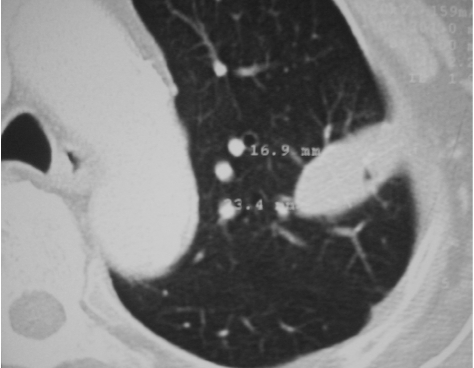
CT scan performed one-month post-RFA. This is a typical appearance of an oval area of coagulation necrosis scarring following RFA. Note that the area of scarring is larger than the original lesion, indicating a positive outcome.
